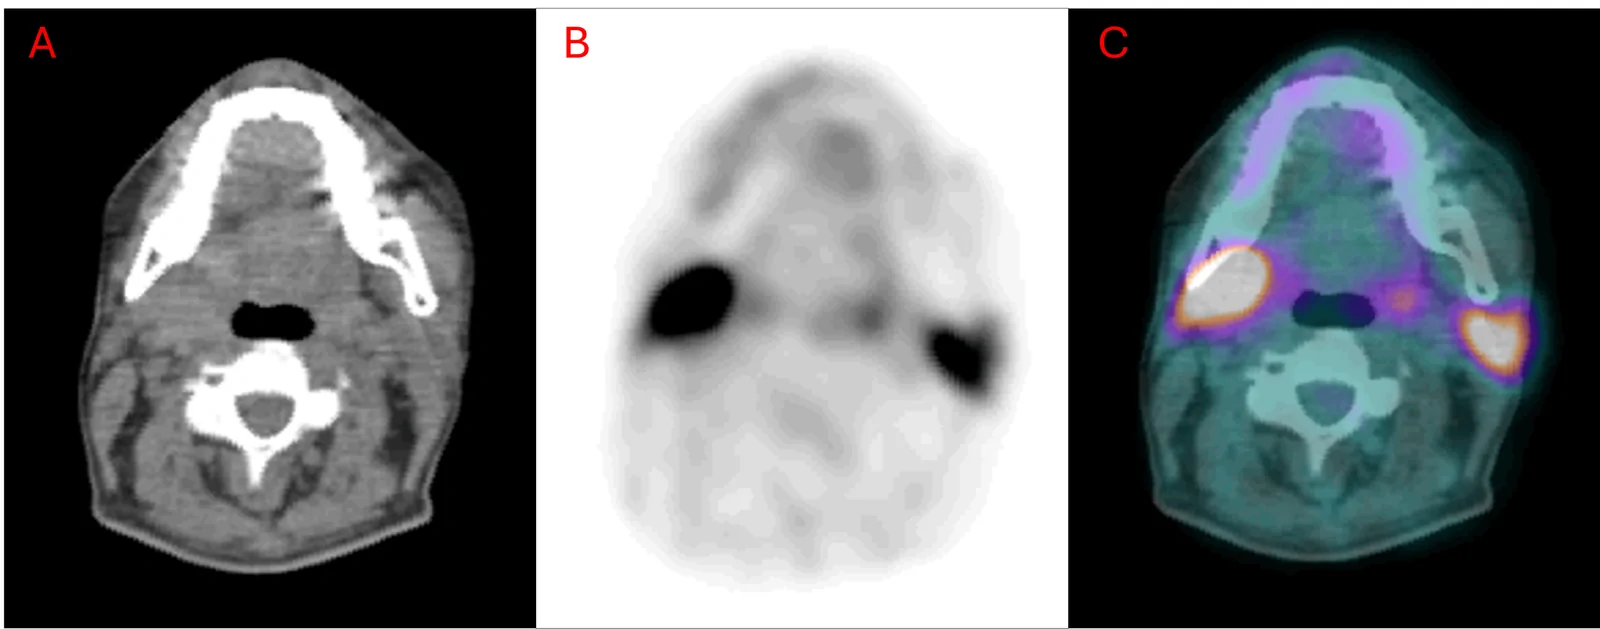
Salivary gland findings on 18F-fluorodeoxyglucose PET/CT. (A) Axial CT image at the level of the salivary glands. (B) Corresponding axial grayscale PET image. (C) Fused axial PET/CT image showing increased ^18^F-FDG uptake in the submandibular and parotid glands, compatible with inflammatory salivary gland involvement.
